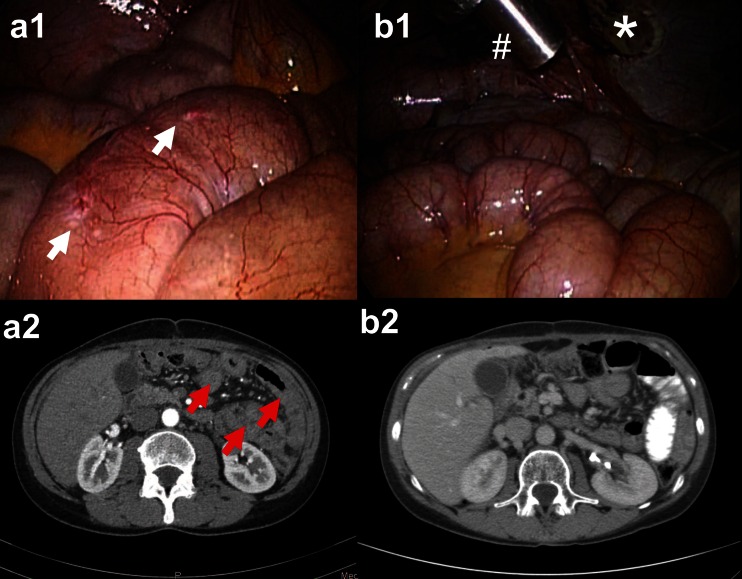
Fifty-one years old female patient after R1 gastrectomy, postoperative chemotherapy (FLOT), and radiochemotherapy (5-FU) for GC, intestinal type, pT3 pN2 pM1 (per). Videolaparoscopy (*a1*) and CT scan (*a2*) at PIPAC#1 showing multiple small bowel involvement (*white arrows*) and radiological diffuse small bowel thickening (*red arrows*). At PIPAC # 4, videolaparoscopy shows a complete macroscopic response (*b1*) and CT a complete radiological response according to RECIST 1.1 criteria (*b2*). *Number sign*: micropump placed into the abdomen during laparoscopy. *Asterisk*: local peritonectomy scar. Multiple biopsies confirm major remission with extensive fibrosis and isolated vital tumor cells. Patient was alive 148 days after 1^st^ PIPAC with a KI of 90 %
